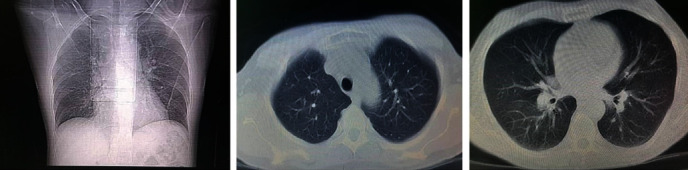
(a) First sonographic image. (b) Second sonographic images.
